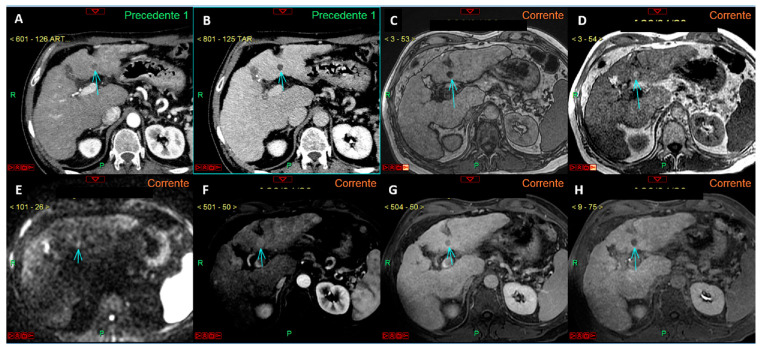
CT images in the arterial (A) and delayed (B) phases show a lesion in the segment III in a cirrhotic liver (arrows in (A,B)), which cannot be accurately characterized as HCC according to EASL guidelines. In the MRI study with a hepatospecific contrast agent (arrows in (C–H)), the lesion exhibits typical features of HCC according to EASL guidelines: arterial phase hyperenhancement (F) associated with contrast media wash-out in the venous phase (G). The lesion also demonstrates additional malignant features, such as signal restriction on diffusion-weighted imaging at b-value 800 (E) and hypointensity in the hepatobiliary phase (H). According to Jiang et al. (see reference no. [31], the lesion shows homogeneous intratumoral fat, as evident from the uniform signal drop in the T1 out-of-phase sequence (C) compared to the T1 in-phase sequence (D): this feature suggests a more favorable prognosis.
